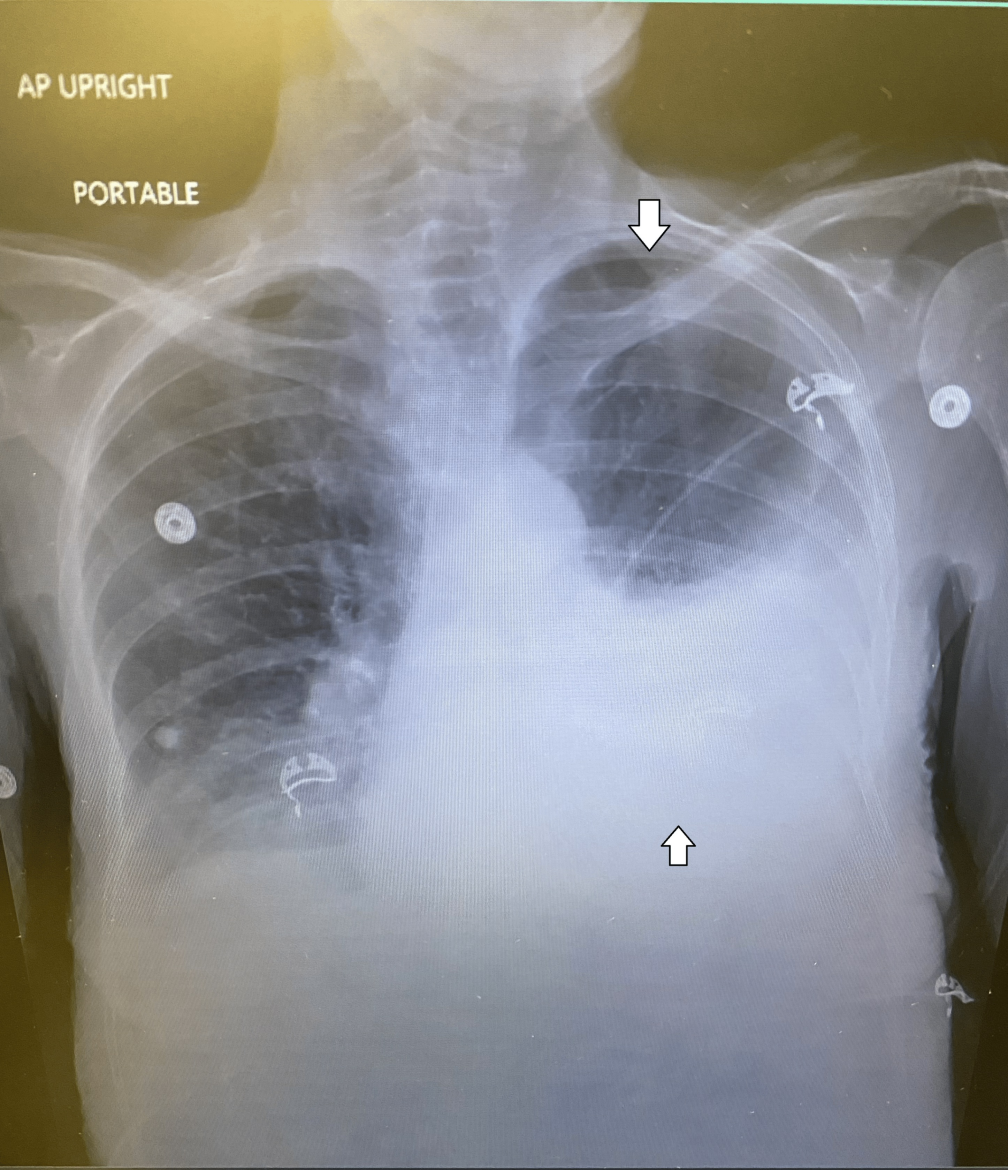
Portable chest X-ray view post-thoracentesis.
